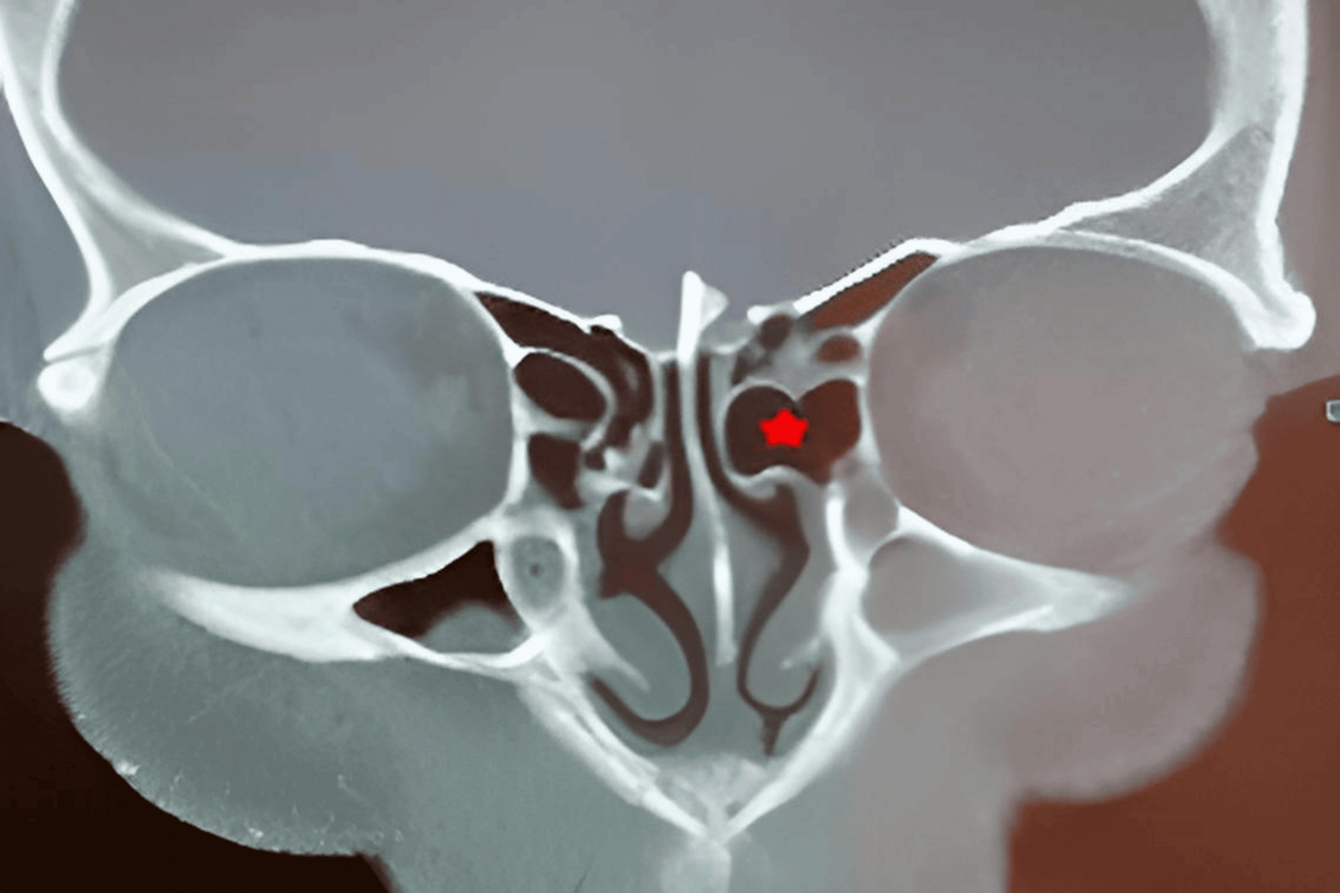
Non-injected coronal section CT scan of the facial mass: left antrochoanal polyp with homolateral hyperpneumatized ethmoid bulla (red star)
